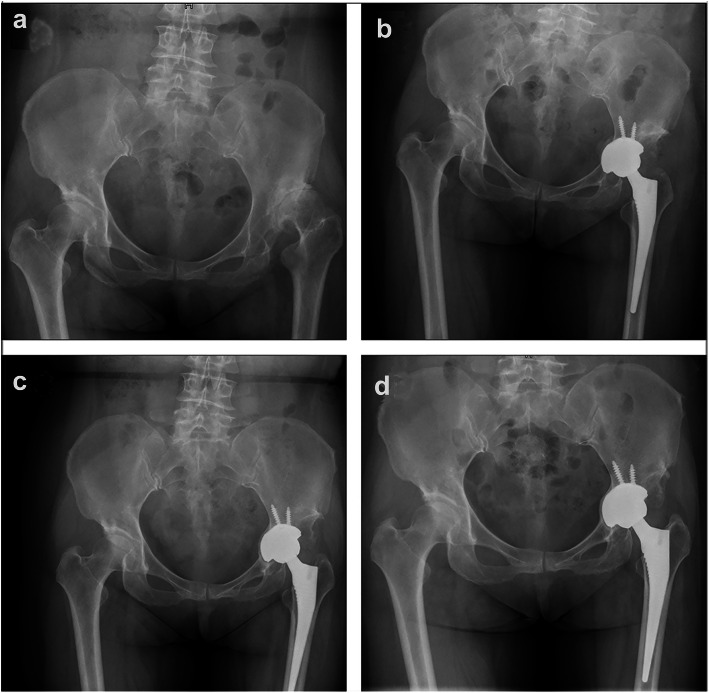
Case presentation of controlled fracture of the medial wall for THA. a A 54-year-old woman was diagnosed with DDH Crowe III on the radiograph of the pelvis preoperatively. b The radiograph of the pelvis after surgery immediately showed the rate of cup coverage was 86%, the rate of medial protrusion was 52%, and the leg-length discrepancy was 0.38 cm. c The radiograph of the pelvis at 1-year follow-up showed the medial wall was a bony union and no aseptic loosening of the component. d The radiograph of the pelvis at 76-month follow-up showed no aseptic loosening and migration of the component
